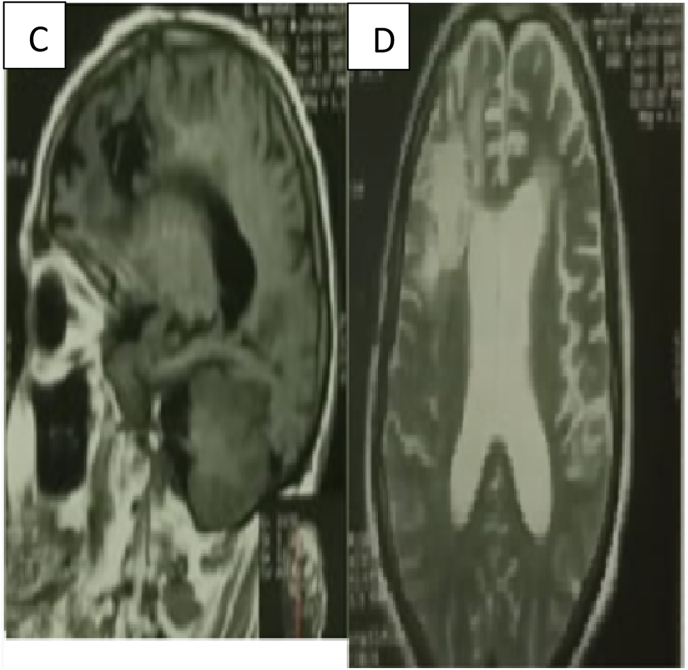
MRI: D: axial image T2, C: sagittal image. no signs of tumor.
